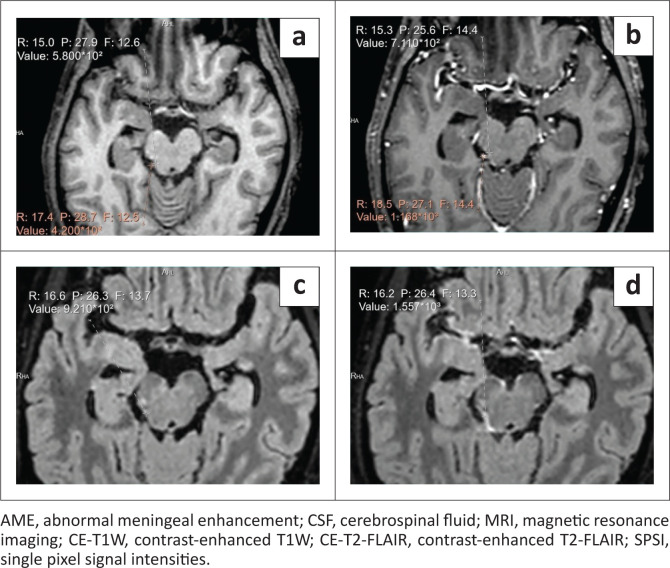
Axial MRI of a 45-year-old male patient with CSF proven meningitis showing placement of the the region of interest (ROI) and measurement of SPSI. The values in white represent meningeal SPSI and the values in red represent vascular SPSI. (a) Pre-contrast T1W image. (b) CE-T1W image where AME is inconspicuous. Single pixel signal intensities of the meninges (711) are less than the SPSI of the adjacent vessels (1168). (c) Pre-contrast T2-FLAIR image. (d) CE-T2-FLAIR image where AME is clearly visualised. Single pixel signal intensities of the meninges are (1557). No adjacent vessel was visualised in the representative axial section.
